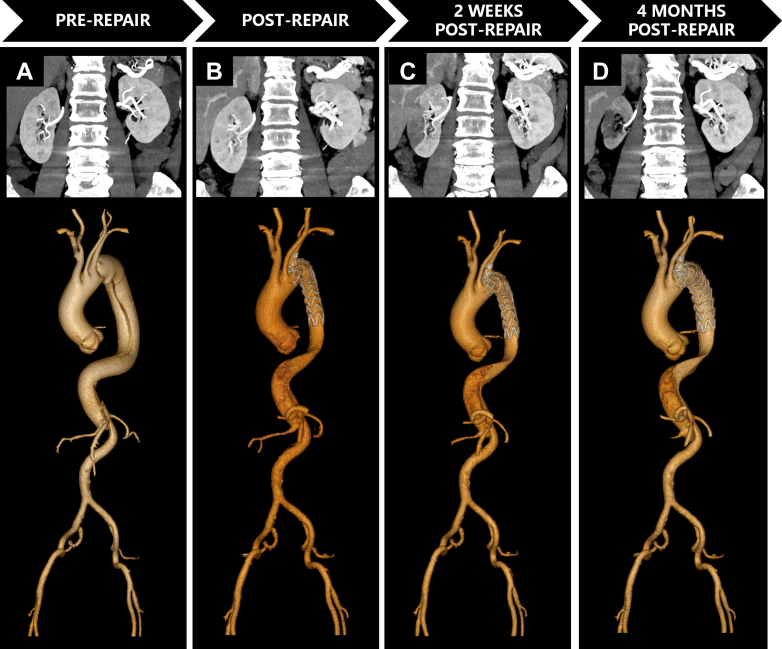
Serial Computed Tomography Angiography Imaging Demonstrating Aortic Evolution and Progressive Renal Atrophy in Patient 1 (A) Preoperative CTA: Stanford type B AD involved right renal artery (RRA) origin; left renal artery (LRA) originates from true lumen (TL) with preserved bilateral cortical enhancement and normal corticomedullary differentiation. (B) Immediate post-repair CTA: Patent RRA with adequate contrast opacification and maintained renal volumes. (C) Two-week CTA: Persistent RRA opacification but new segmental cortical hypoperfusion with ostial stenosis. The false lumen (FL) volume remains stable. (D) Four-month CTA: Critical RRA stenosis, significant renal volume loss (73% of preoperative), and near-absent parenchymal perfusion—classic.
